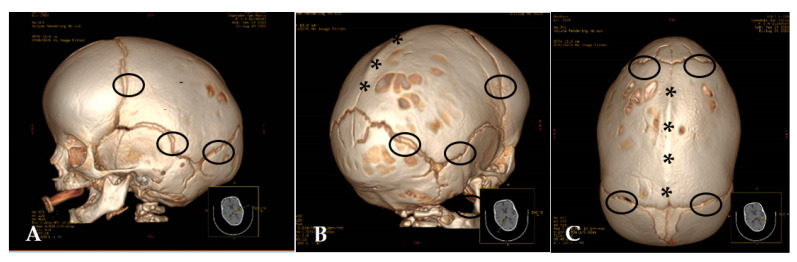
(A–C) CT 3D-Reconstructions (A) Scaphocephaly with patent sutures (ring); (B,C) Patent sutures (ring) and synostotic sagital suture (asterisks).
